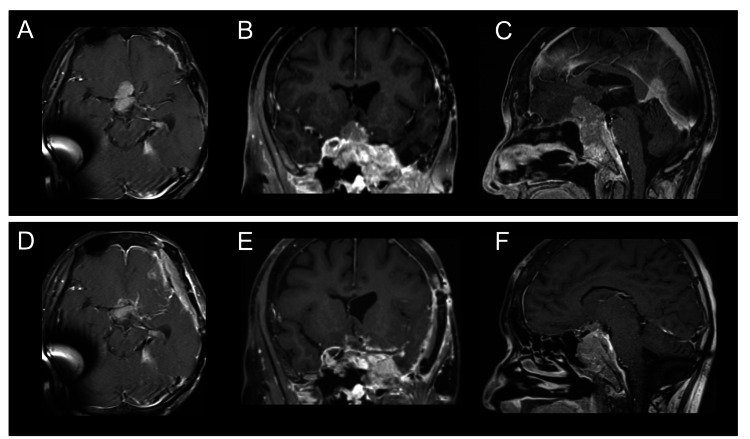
Pre and postoperative contrast-enhanced T1-weighted images Preoperative axial (A), coronal (B), and sagittal (C) sections of contrast-enhanced T1-weighted images revealed a skull base tumor extending to the suprasellar region. Postoperative axial (D), coronal (E), and sagittal (F) sections of contrast-enhanced T1-weighted images showed partial removal of the tumor around the optic nerves.
